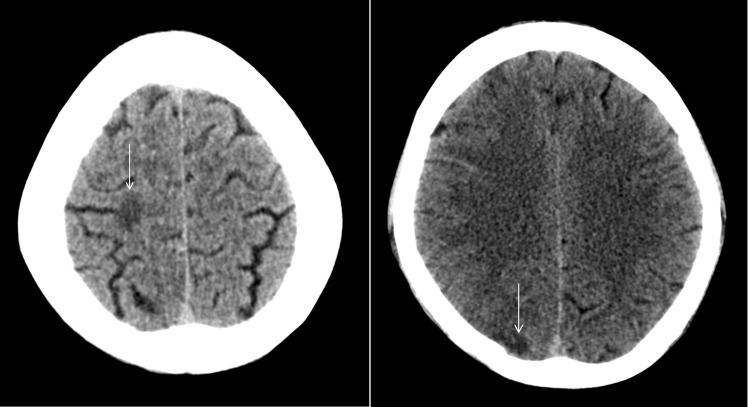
The Left part is showing axial CT scan of the brain which shows right parietal subcortical white matter hypodensity (arrow). Right: Fig Axial CT scan of the brain shows right frontal subcortical white matter hypodensity (arrow).
